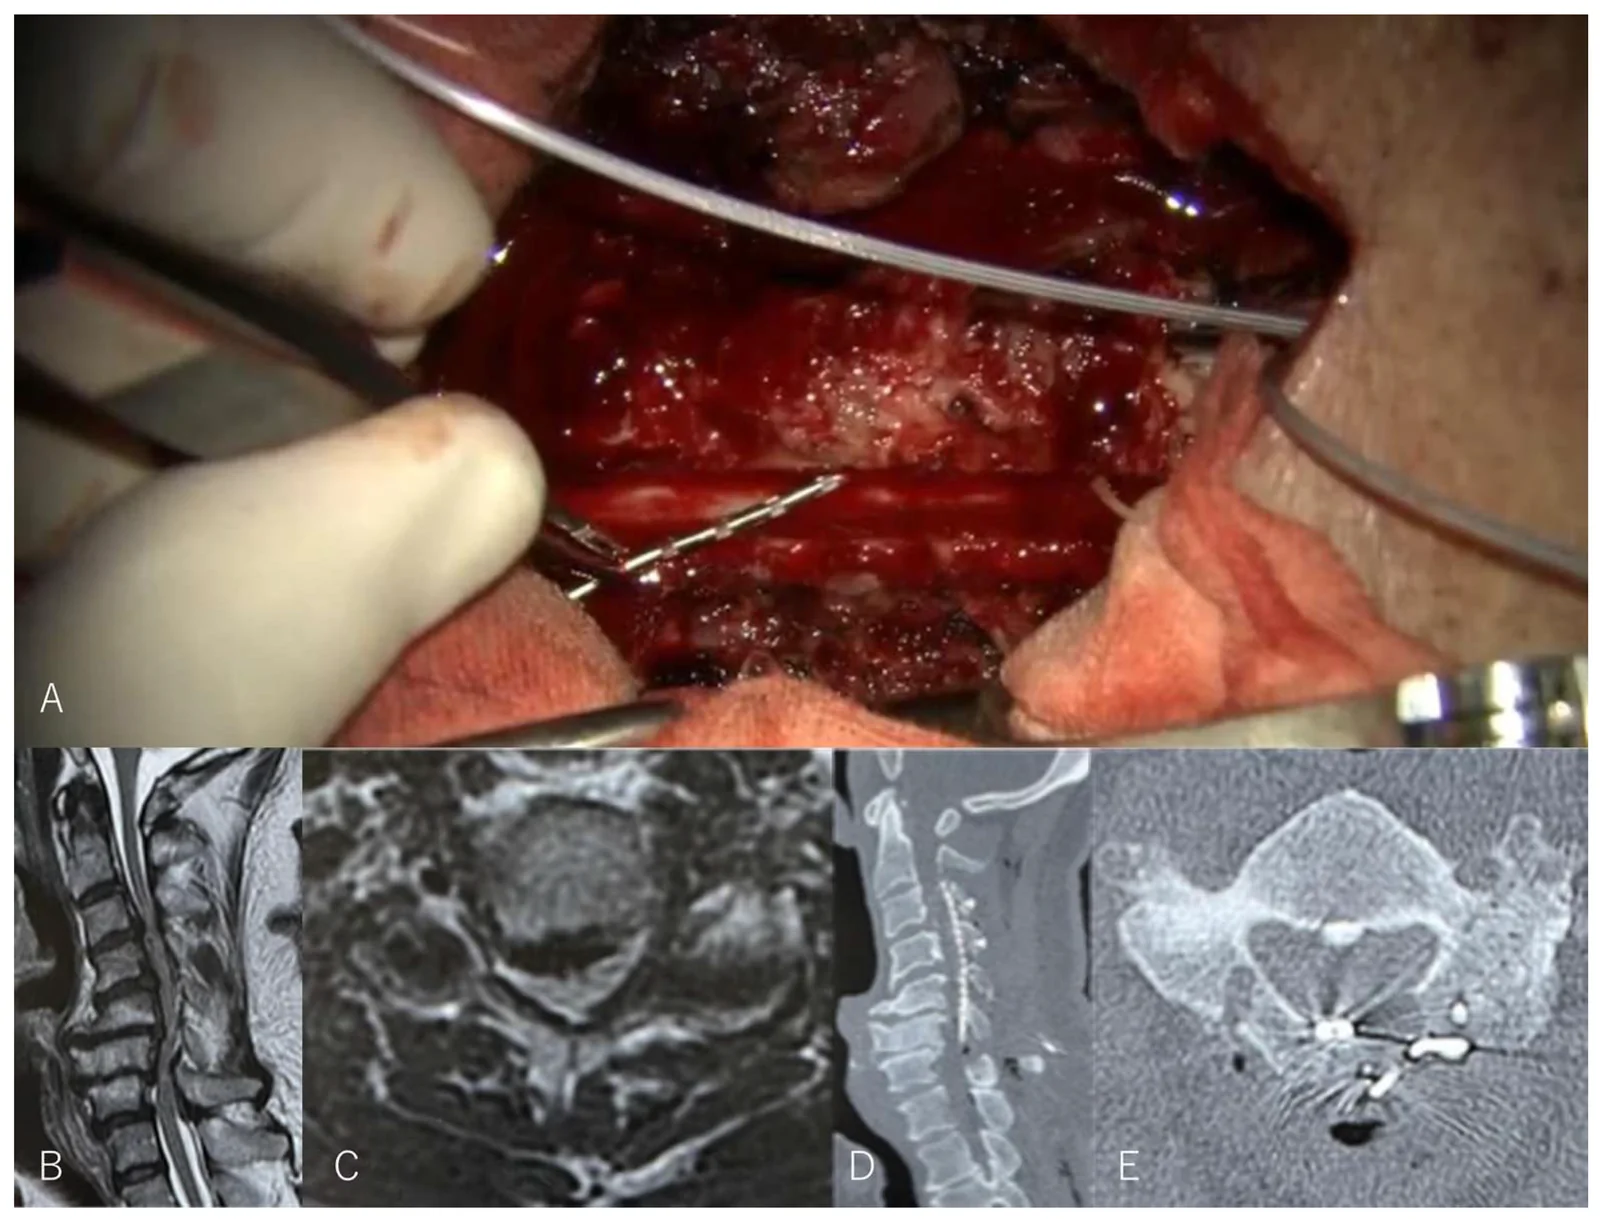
Intraoperative and radiographic findings in the representative case (Patient 3). (A) Intraoperative photograph following C2-7 open-door laminoplasty, with the head to the left and the caudal end to the right. After spinal cord decompression, two SCS leads were advanced and positioned in the epidural space by sliding them in a cephalad direction. (B) Preoperative T2-weighted MRI (sagittal view) and (C) preoperative T2-weighted MRI (axial view) demonstrating multilevel spinal cord compression due to cervical OPLL. (D) Postoperative CT (sagittal view) and (E) postoperative CT (axial view) confirming bilateral SCS lead placement at the intended levels.
